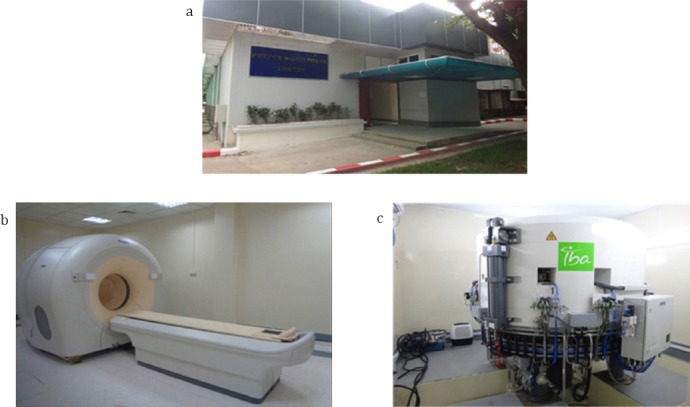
Positron emission tomography–computed tomography and cyclotron. a: PET CT and Cyclotron Center, b: 1.2.2015 PET CT Gemini, TOF,16 slices, c: 1.3.2016 Cyclotron 18 Mev Iba
